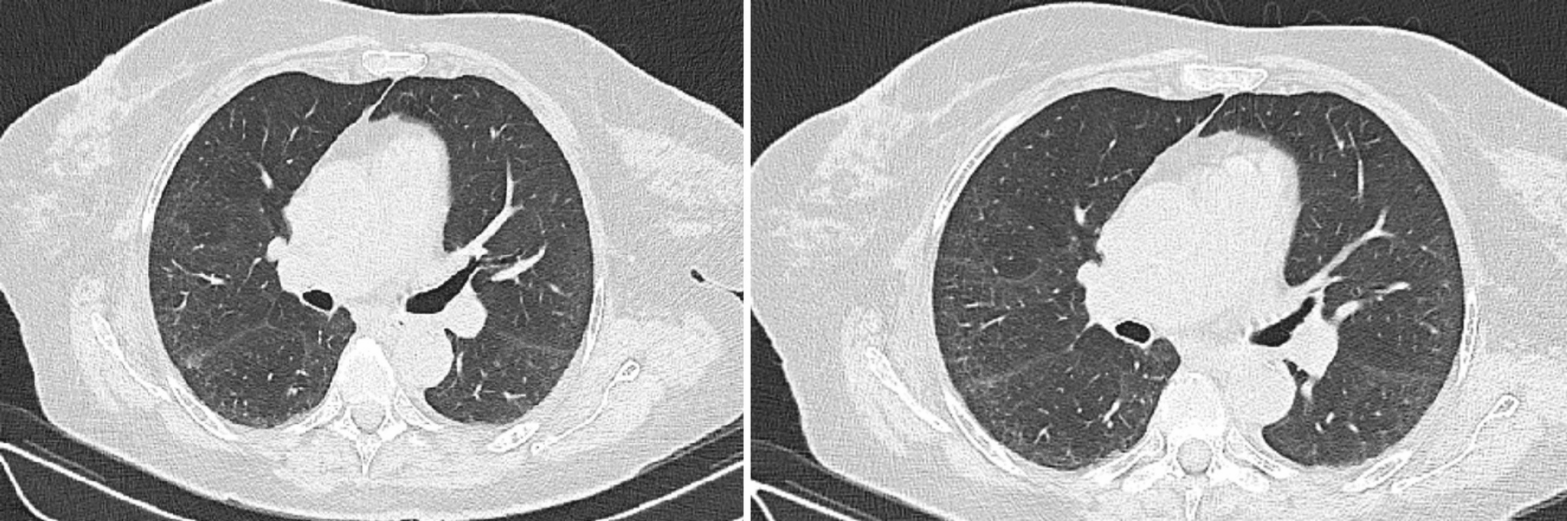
Computed tomography (CT) scan performed at the time of admission
